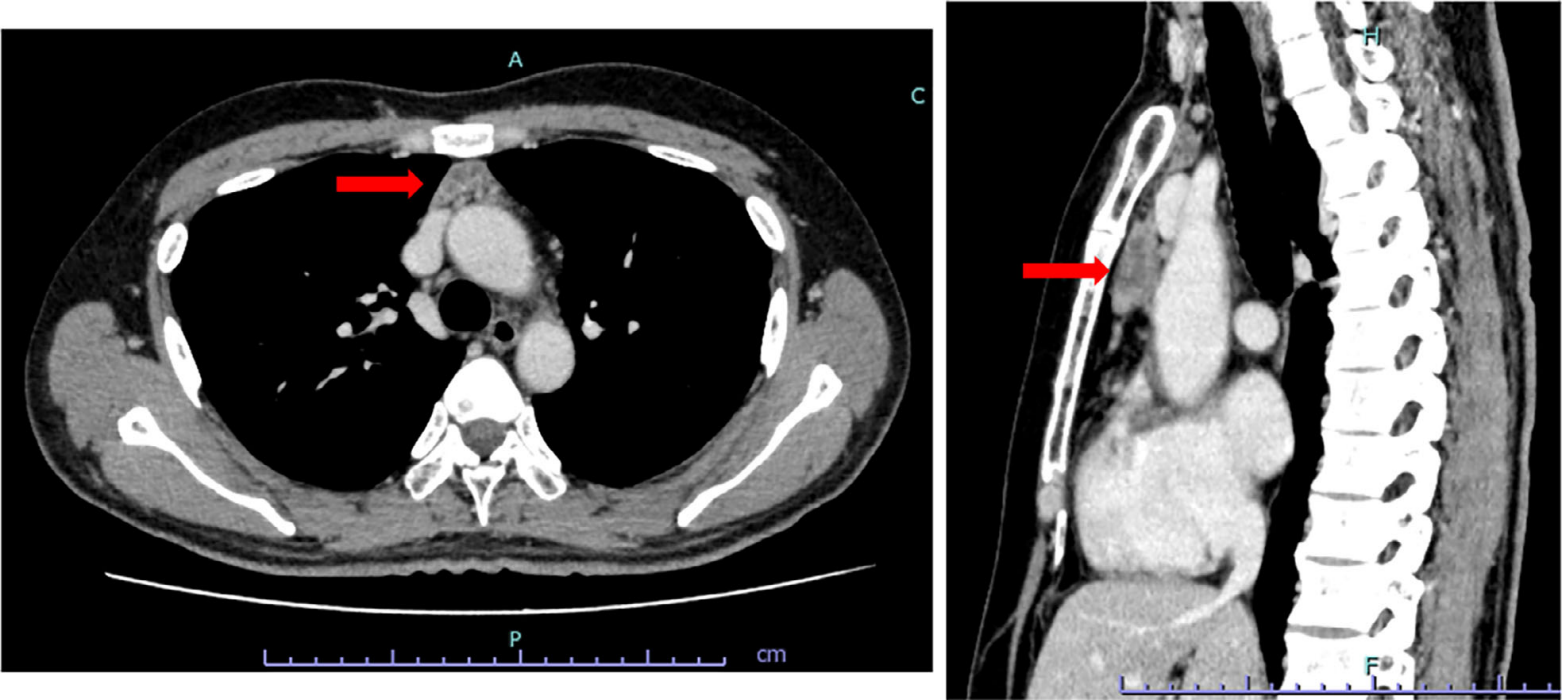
Contrast‐enhanced computed tomography revealed a 4.0 × 2.5 cm mass in the anterior mediastinum with multiple cysts inside, suggesting thymoma, thymic hyperplasia, or lymphoma.
